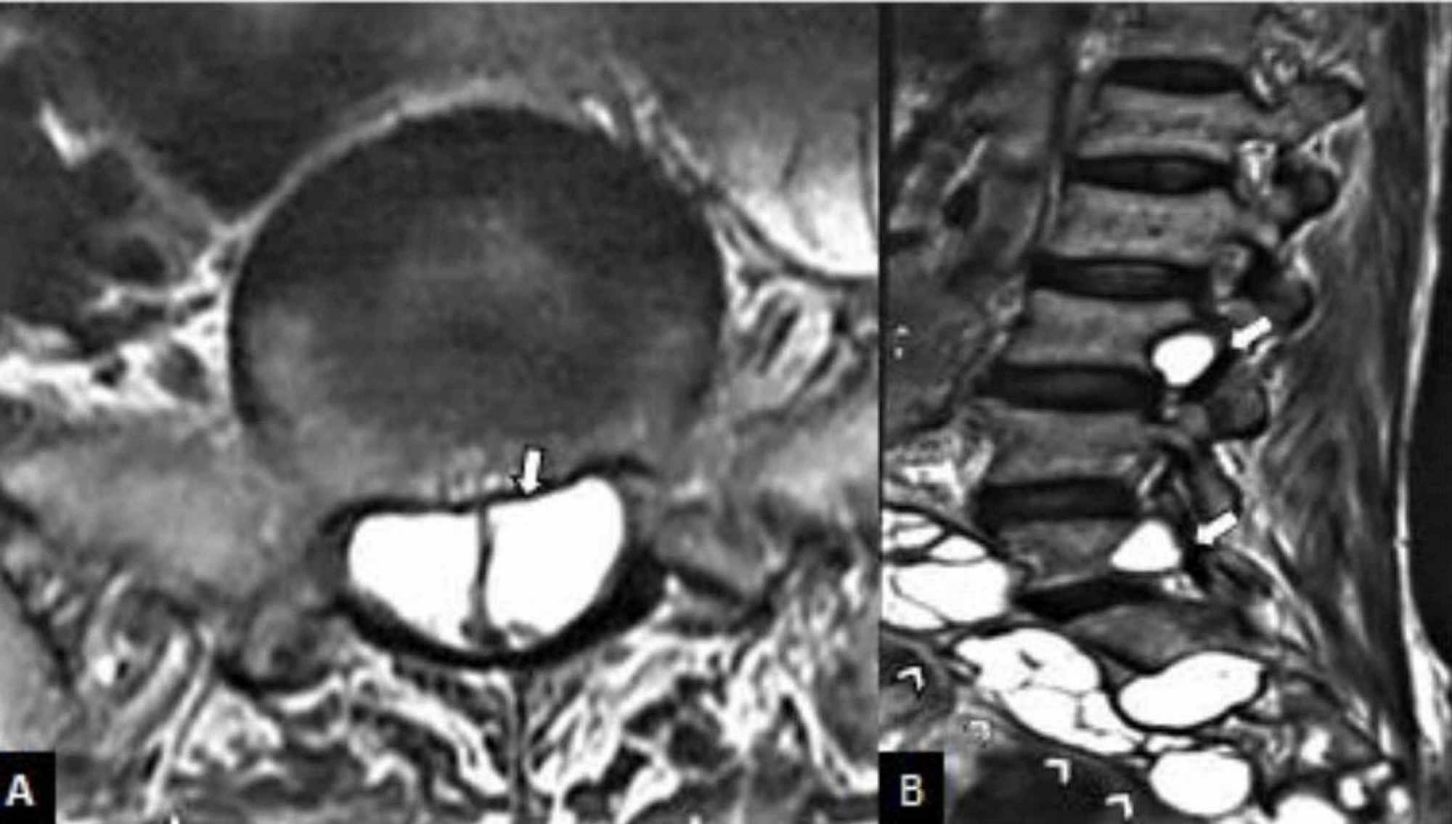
MRI of lumbosacral spine of 36-year-old patient with spinal hydatid cyst A: axial T2WI; B: sagittal T2WI MRI of lumbosacral spine showing multiple intradural (white arrows) and paraspinal hydatid cysts (white arrow heads) MRI: magnetic resonance imagine; T2WI: T2 weighted image
